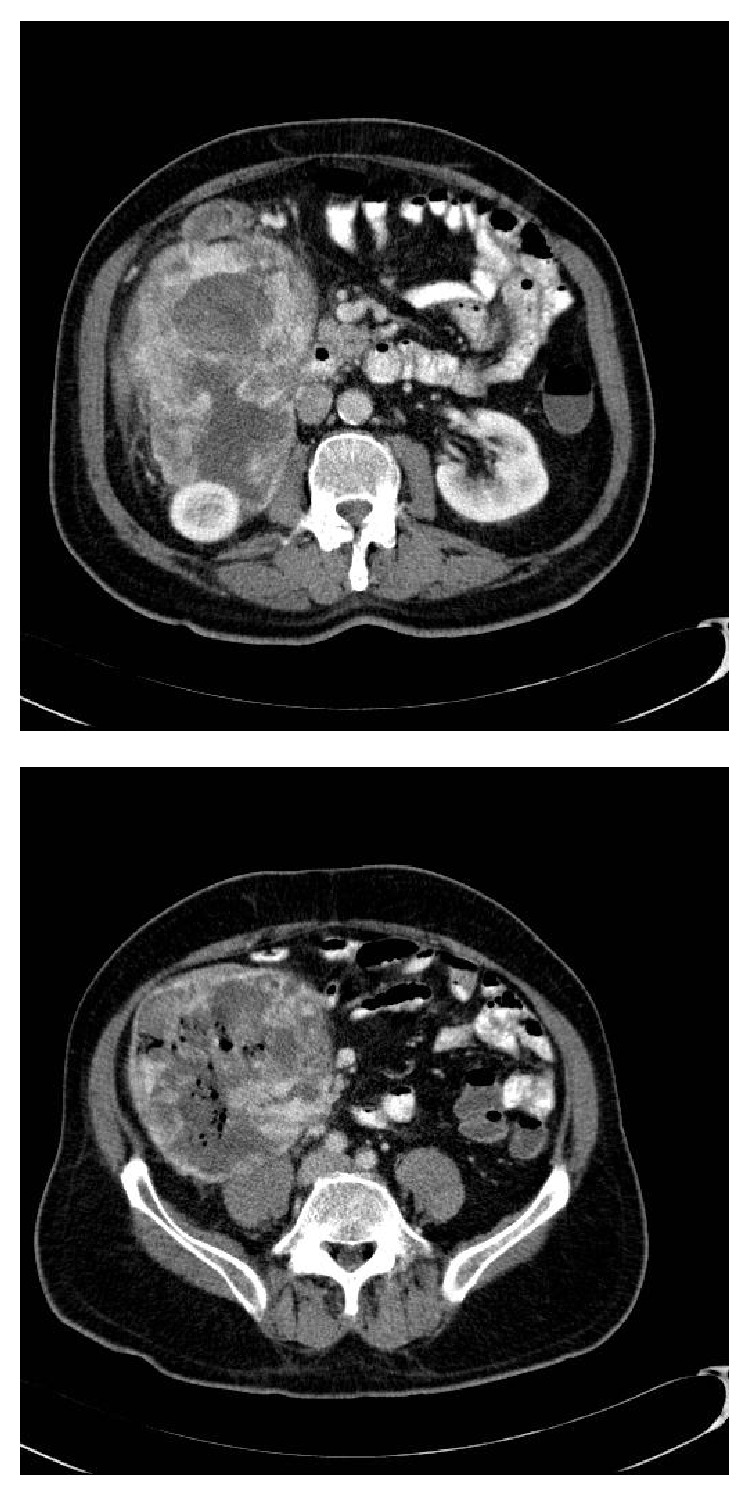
A CT scan shows a heterogeneous, well-circumscribed mass in the right abdominal loin that seems to originate from the hepatic flexure colon and descends towards the anterosuperior iliac spine. Note the presence of air bubbles due to the colonic invasion.
